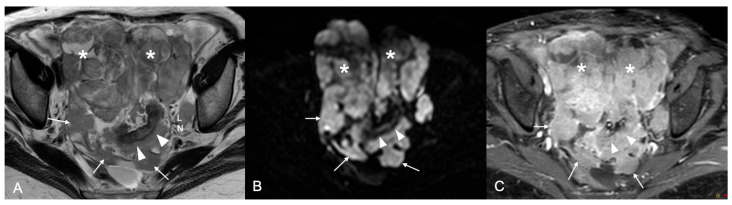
Axial MR images of the pelvis. (A) T2W image shows large, confluent mixed cystic-solid ovarian masses (asterisks) along with diffuse peritoneal involvement of the pelvic peritoneum (arrows) and large deposits on the sigmoid bowel serosa (arrowheads). Enlarged left internal iliac node also present (LN). The ovarian as well as the peritoneal masses demonstrate high signal intensity on the b 1200 DWI image (B), indicative of restricted diffusion, and intense enhancement on the T1W sequence post-contrast with fat saturation (C).
